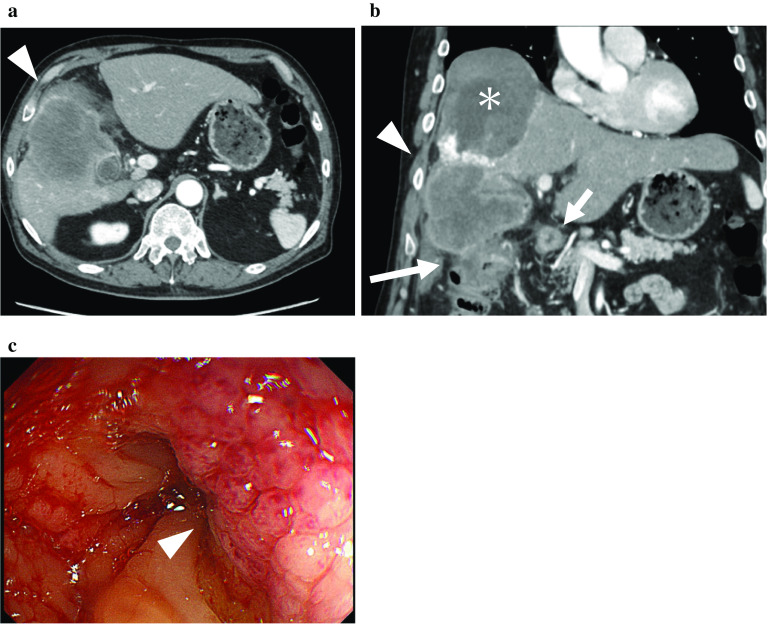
Preoperative imaging findings. a Preoperative abdominal computed tomography scan. Axial view (left) and coronal view (right) showed an enlarged low-attenuation mass that was 80 mm in diameter. The mass involved the S5 segment of the liver (arrowhead) and the hepatic flexure of the transverse colon (large arrow). A nodule was observed in the transverse mesocolon (small arrow). * indicates cavernous hemangioma. b Preoperative colonoscopy. An elevated lesion was detected (arrowhead) on the transverse colon, which was a result of compression from the tumor
